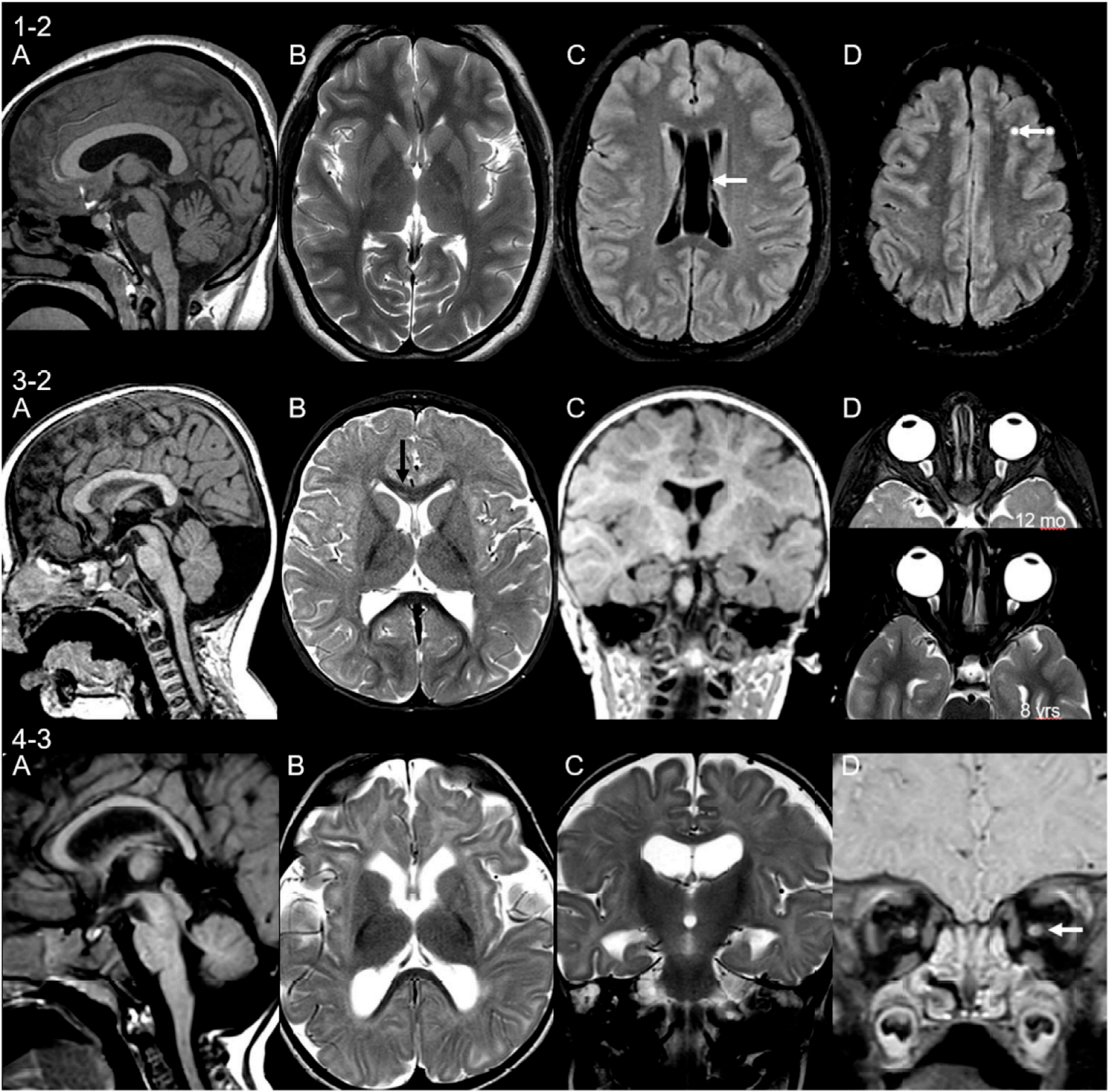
Neuroimaging findings in three unrelated patients. MRI of patient 1-2 at age 15 years shows (A) thick corpus callosum and mild cerebellar hypoplasia, (B) small basal ganglia, (C) cavum septum pellucidum et vergae (arrow), and (D) non-specific punctate white matter lesions on fluid-attenuated inversion recovery (FLAIR) sequences (arrow). Patient 3-2 scanned at 12 months of age shows (A) retrocerebellar cyst and thin cervical cord, (B) signal change of the genu of corpus callosum (immature myelin, black arrow) and small lentiform nucleus, (C) malrotation of hippocampi, and (D) asymmetry of the globes, more prominent on follow-up (lower image). Patient 4-3 at 7 months of age shows (A) small cerebellum and pons and drooping splenium, (B) microcephaly with volume loss of the white matter, delayed myelination, and volume loss of the lentiform nucleus, (C) malrotation of hippocampi, and (D) volume loss of the optic nerves.
